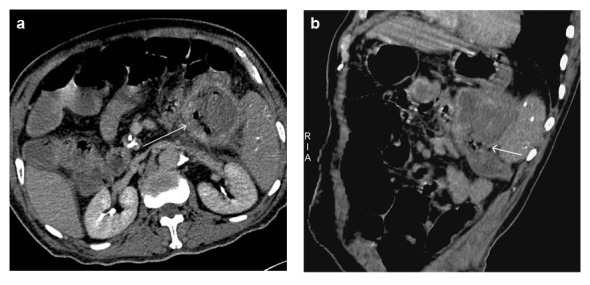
Axial and oblique reconstructed CT images showed ill-demarcated margin with splenic flexure of the colon and the presence of air bubbles within pseudocyst, suggesting the communication between pancreatic pseudocyst and colon.
